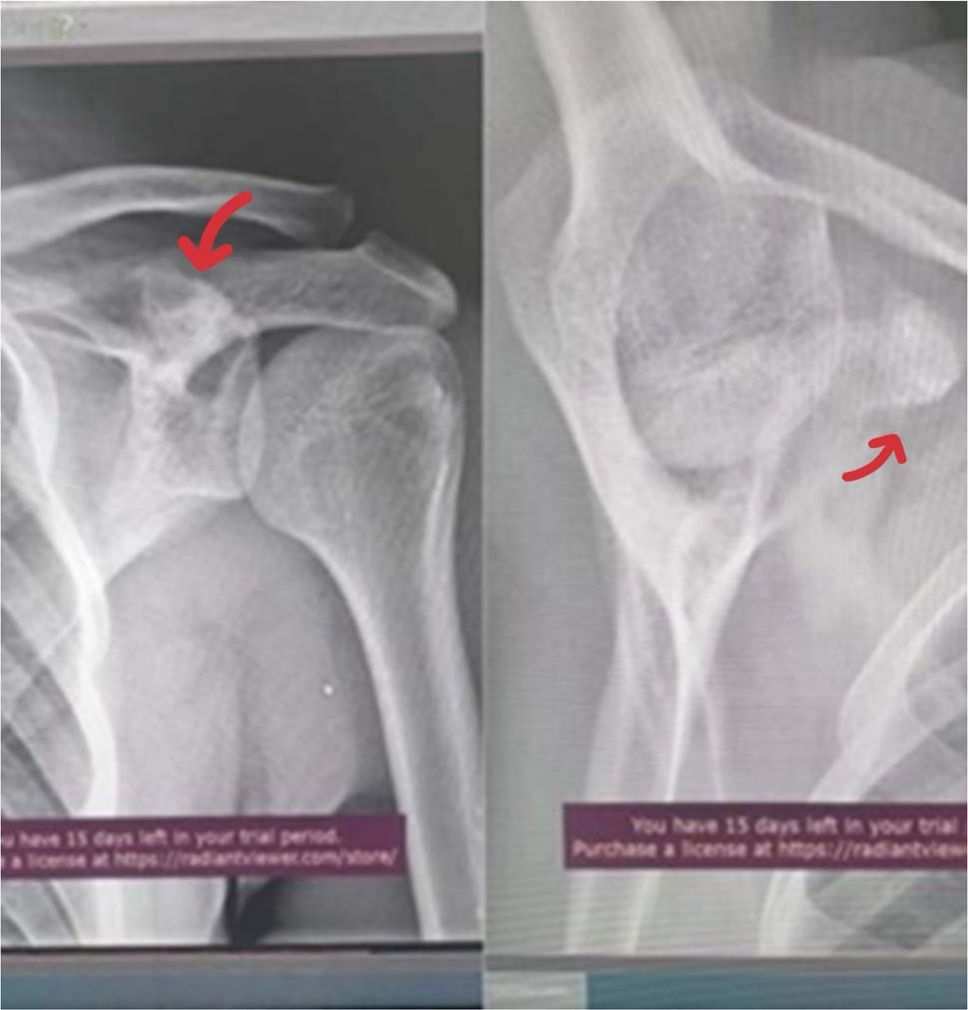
AP radiograph of the left shoulder shows a suspension lesion in the coracoid process of the scapula (indicated by arrow).
